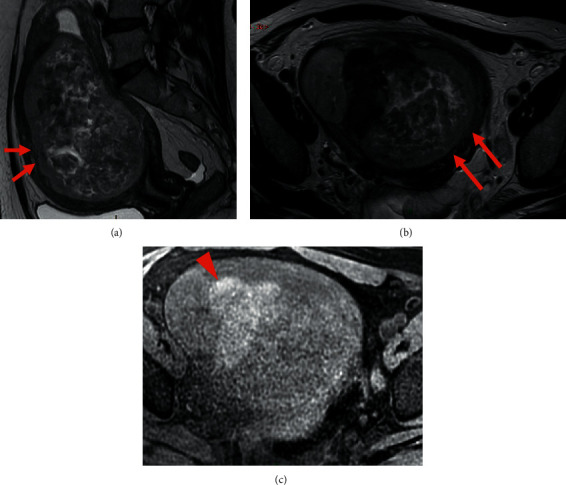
Pelvic MRI of leiomyosarcoma: (a, b, c) sagittal (a) and axial (b) T2 and axial (c) T1 Fat-Sat WI showing a large uterine tumor developed within the myometrium, showing heterogeneous intermediate T2 signal with irregular margins (arrows) and intratumoral hemorrhage in high T1 Fat-Sat WI signal (arrowhead) (c).
